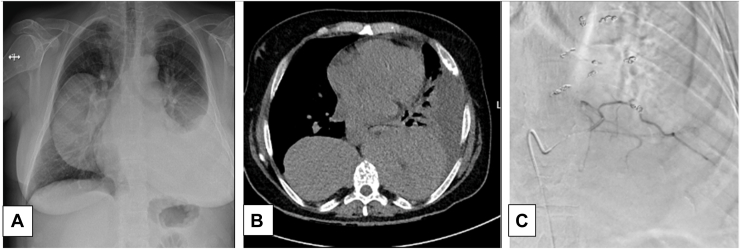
(A) Chest radiograph showing left-sided hemothorax and bilateral extramedullary hematopoiesis masses. (B) Computed tomography scan of the thorax showing the bilateral extramedullary hematopoiesis masses and the left-sided hemothorax. (C) Angiography image during embolization of the intercostal arteries with microcoils.
